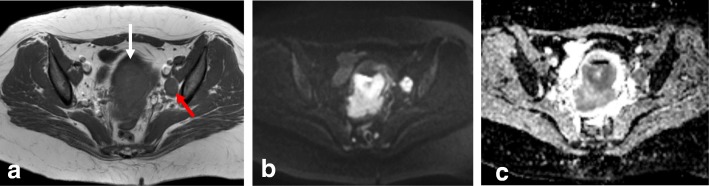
Axial oblique T2W (a) and DWI images (b, c) showing stage IIIC 1 endometrial cancer (white arrow) with metastatic left obturatory lymph node (red arrow)
